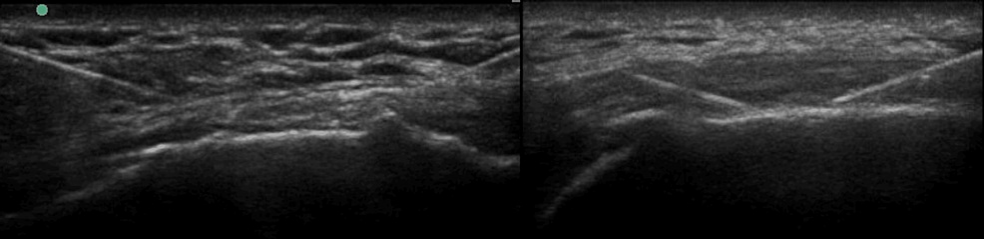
The image depicts the needle positioning with ultrasound guidance in cases three and five, respectively. The green dot indicates the cranial side (in both ultrasound pictures).
